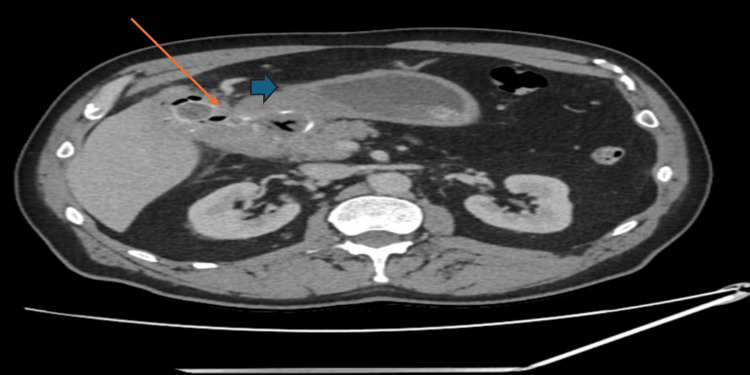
Computed tomography (CT) showing cholecytoduodenal fistula secondary to a chronic calculous CT scan demonstrating a 3 cm gallstone in the distended stomach (blue arrow) with pnuemobilia and collapse of the distal bowel loops. The thick-walled gallbladder contains multiple stones (orange arrow). These findings are consistent with Bouveret's syndrome.
